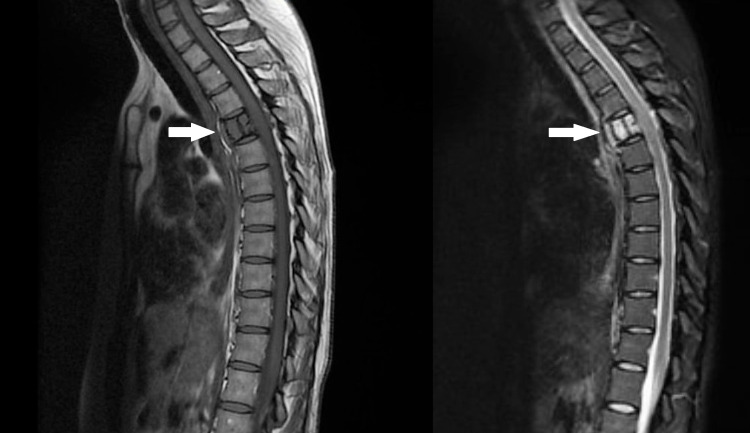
MRI of the spinal column showing a lesion centered on the vertebral body of T5 (arrows)
